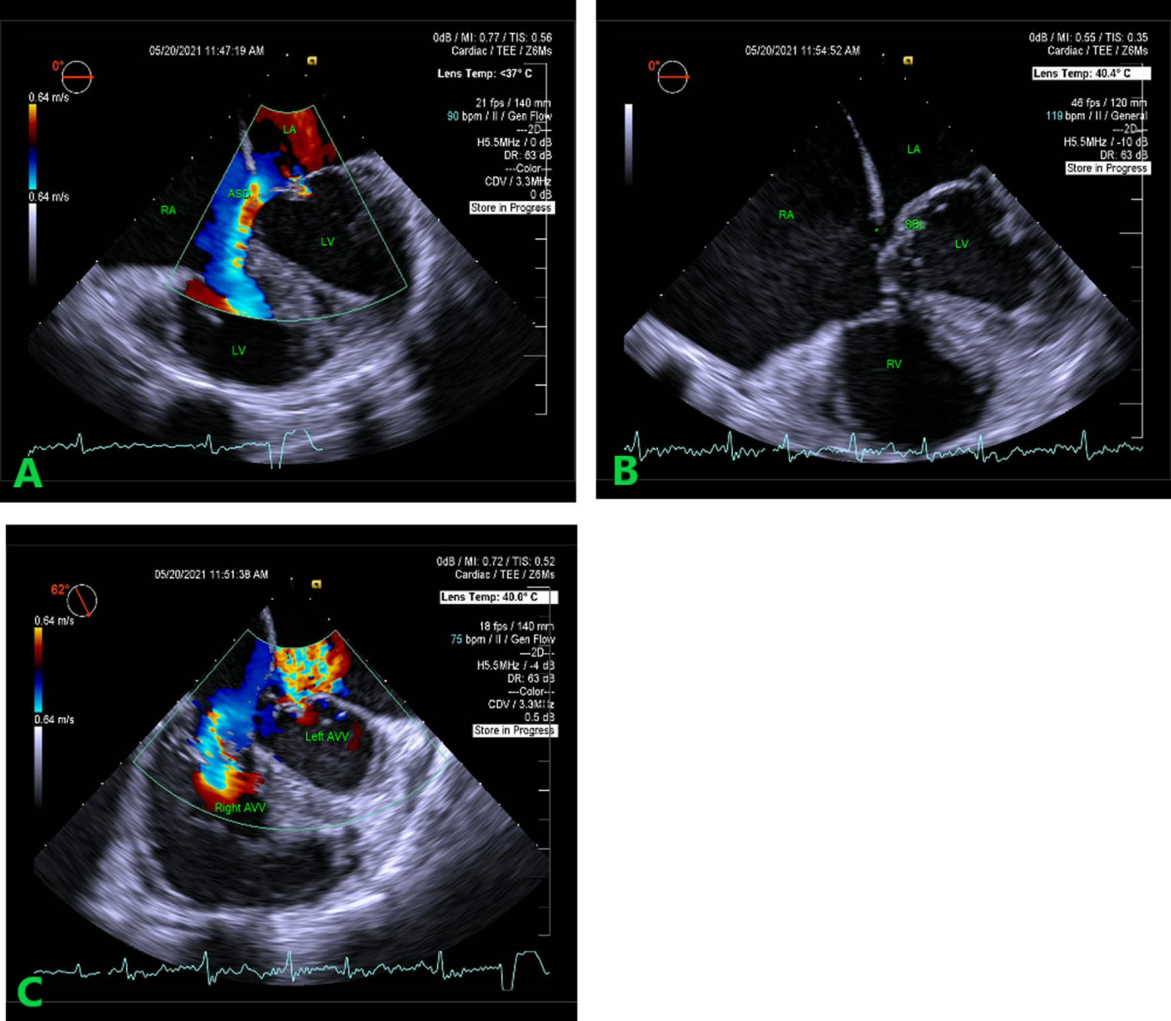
A AVSD—2D TEE. Primum atrial septal defect with a left-to-right atrial shunt in the color flow mapping. B 2D-TEE. AV septum deficiency and superior bridging leaflet attachment to the interventricular septum. Midesophageal four-chamber view. The primum atrial septal defect is also seen (asterisk). C AV valves regurgitation. Color flow-mapping imaging of the common AV valve presented as severe valvular regurgitation with a higher grade observed on the right component. *AVSD* atrioventricular septal defect, *TEE* transesophageal echocardiography, *AV* atrioventricular
